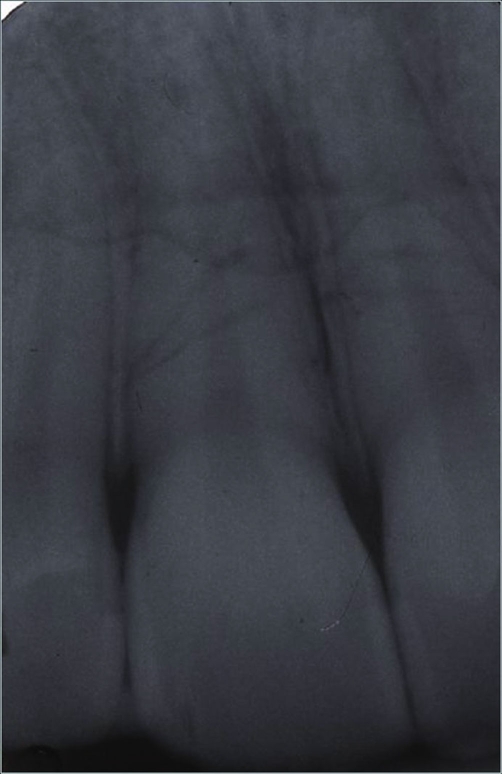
Radiographic control at 4 years.
